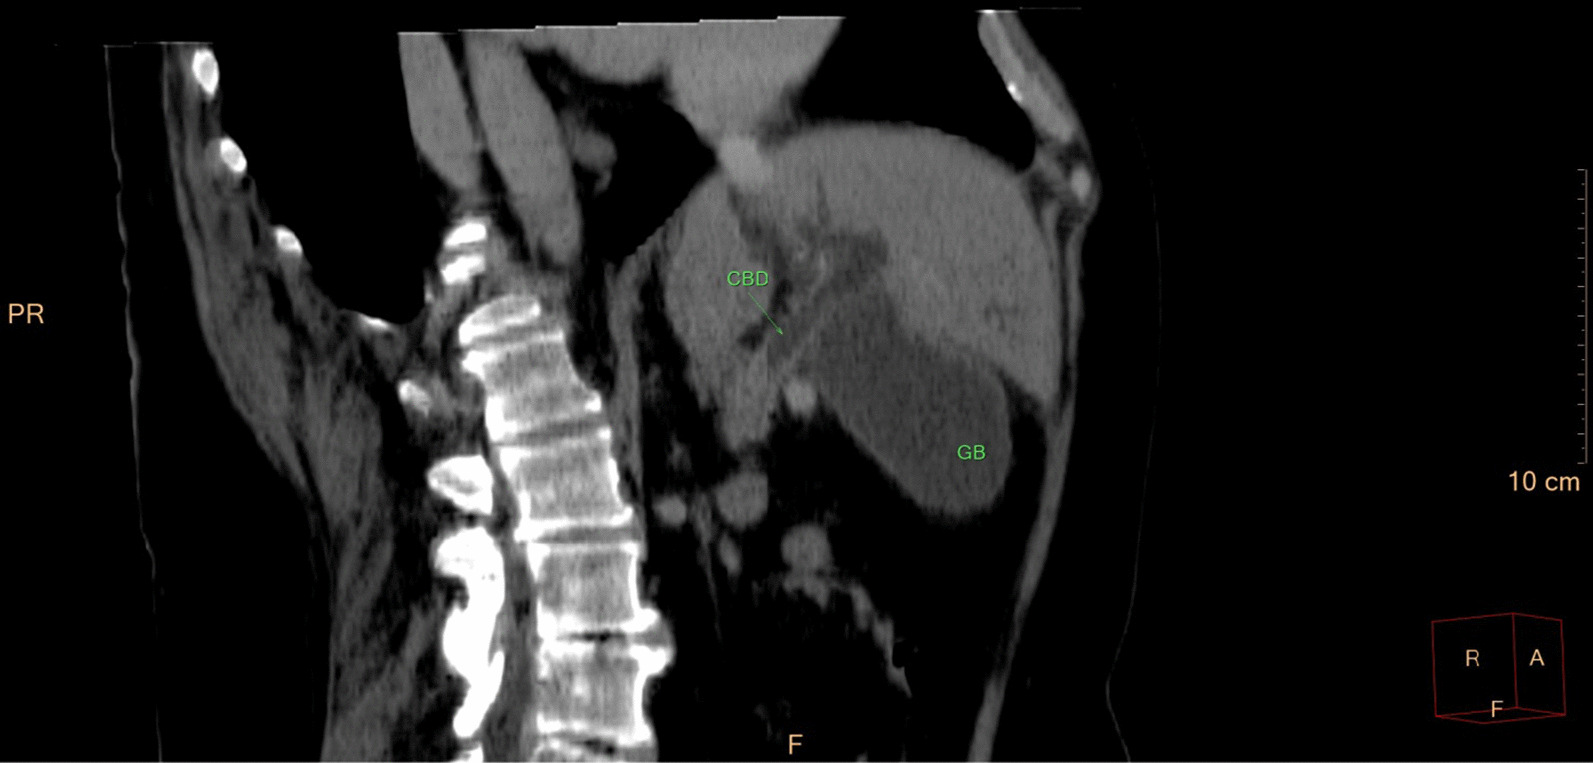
CT – pre-stenotic common bile duct dilatation
